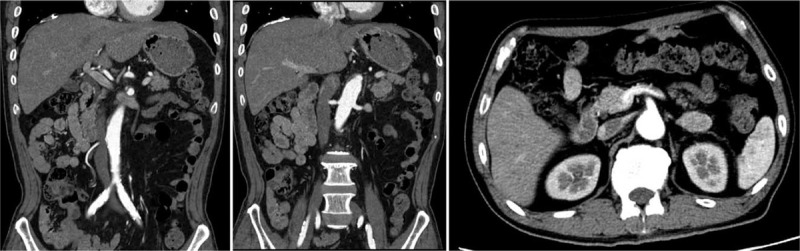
Contrast-enhanced CT on day 44: thrombus nearly completely disappeared. CT = computed tomography.
